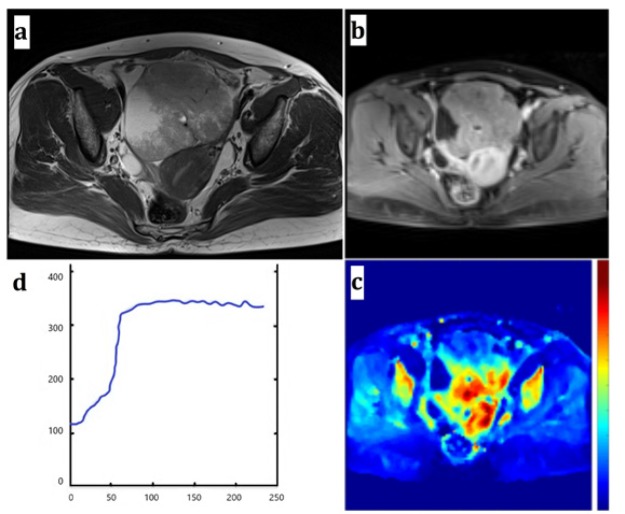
Region of Interest (ROI) drawn on a malignant adnexal mass (an endometrioid carcinoma) (a) Axial T2-weighted image (b) Axial DCE T1-weighted image (c) Ktrans coded color map, ROI was drawn on a solid portion of the tumor. (d) Malignant enhancement curve and pharmacokinetic and semi-quantitative parameters
